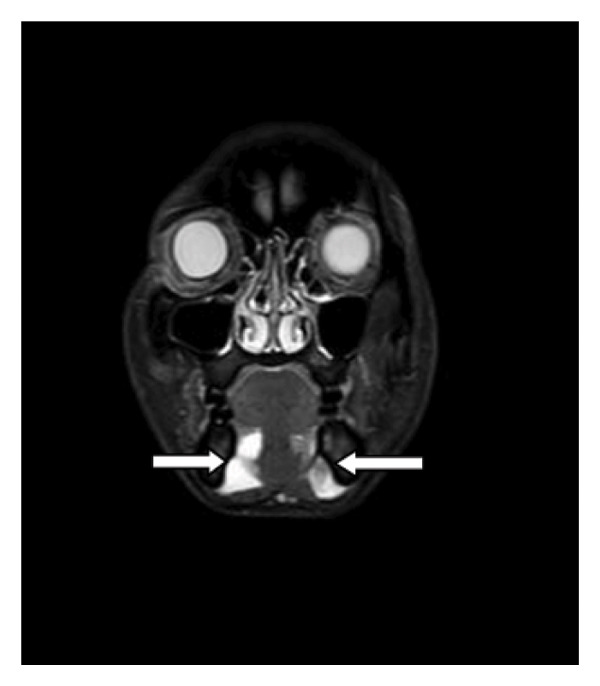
Magnetic resonance imaging of the coronal plane demonstrates bilateral cystic masses adjacent to the mandible, extending into the submandibular space. The masses exhibit well‐defined borders and show no enhancement.
